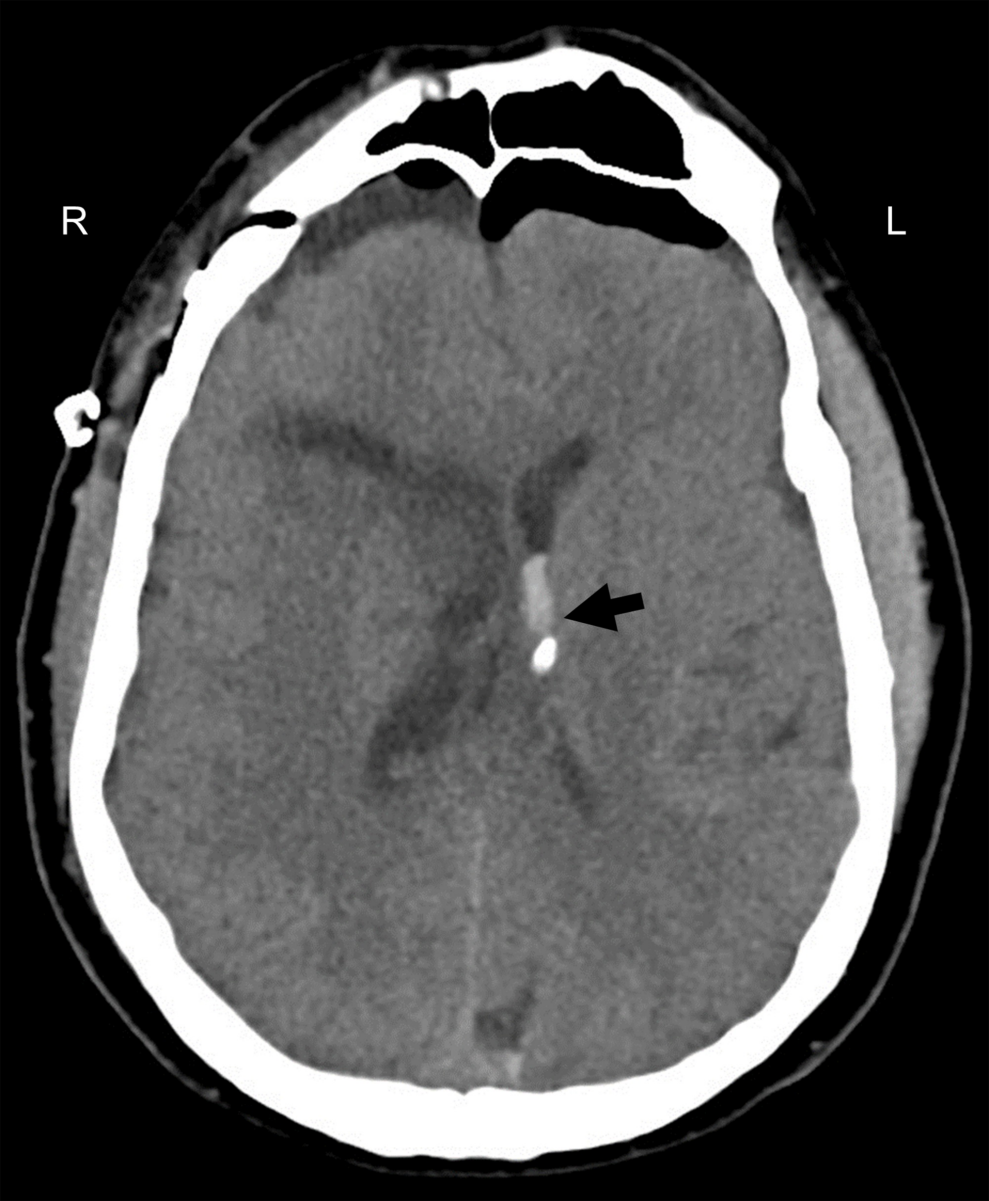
Post-operative head computed tomography (CT). Almost complete removal of the intracerebral hemorrhage and intraventricular hemorrhage from the right lateral ventricle but with persistent clot within the left lateral and third ventricles.
